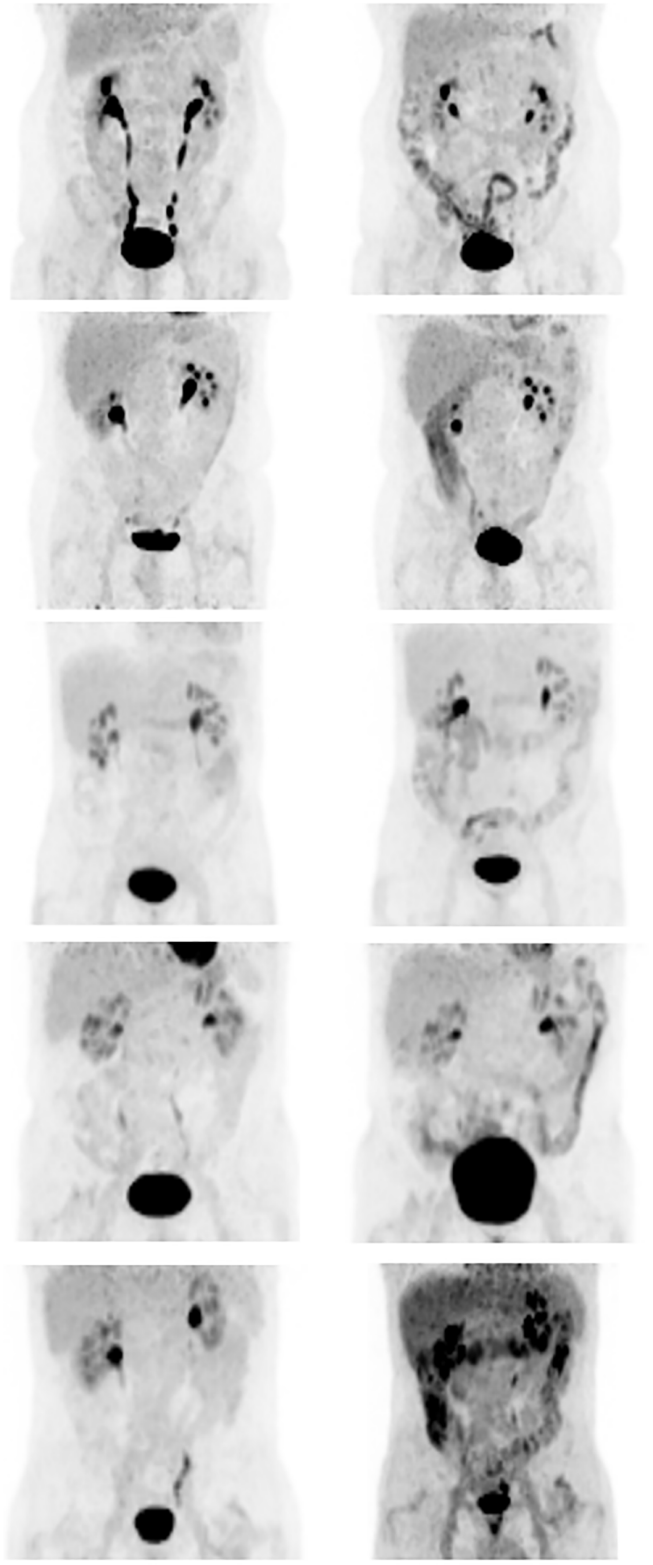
Typical cases. Five typical examples of ^18^F-FDG uptake in the colon before (left panel) and after (right panel) the administration of metformin. Please note the increased ^18^F-FDG uptake in the colon after metformin administration.
